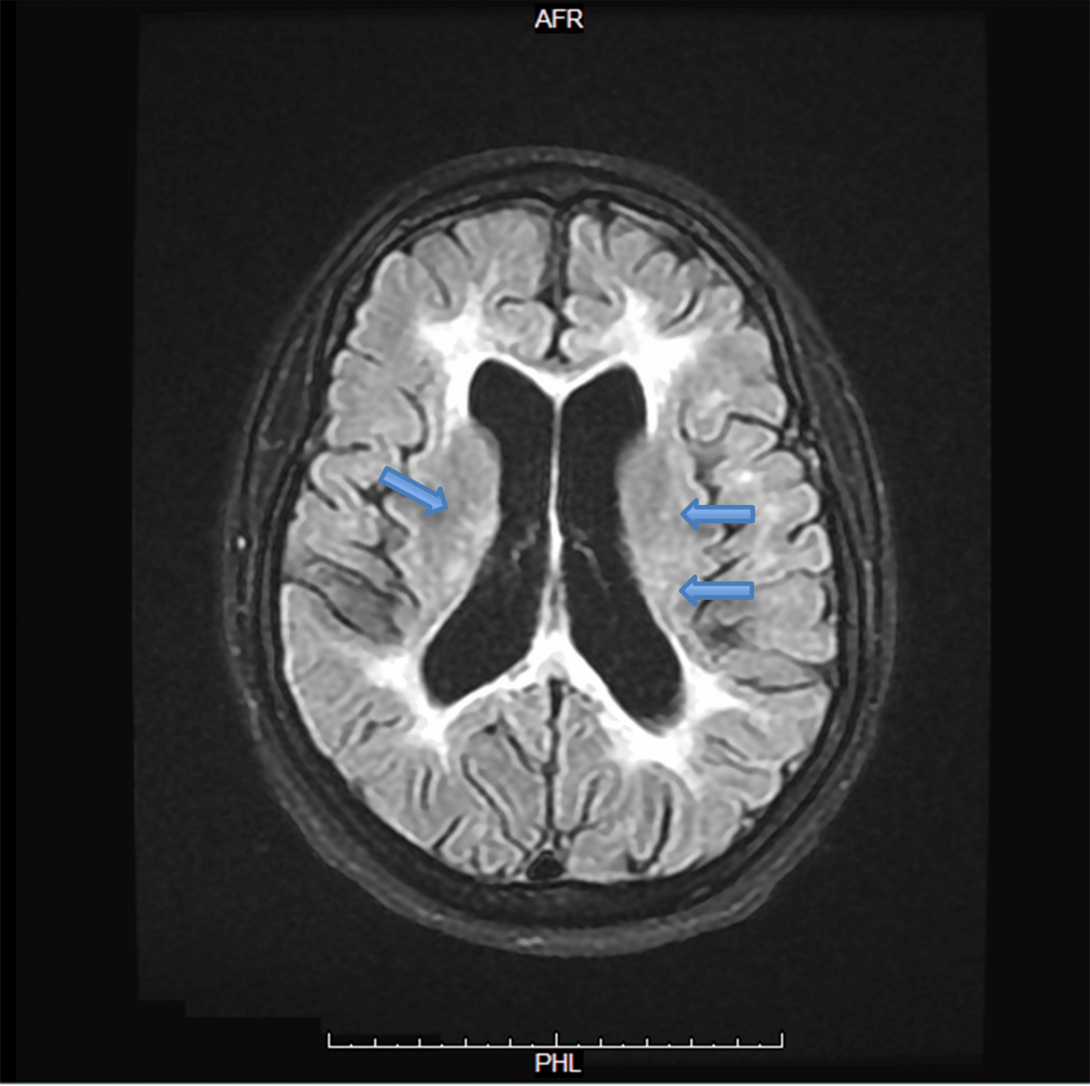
Axial T2 FLAIR MRI of the brain without contrast Axial T2 FLAIR brain MRI shows hyperintense white matter lesions seen around the periventricular regions bilaterally (blue arrows), extending along the lateral ventricles. The distribution appears symmetrical. The ventricles themselves look mildly prominent, but there is no evidence of acute hemorrhage, mass effect, or midline shift. The cortical sulci and gray–white matter differentiation are preserved MRI: magnetic resonance imaging; FLAIR: fluid attenuated inversion recovery
